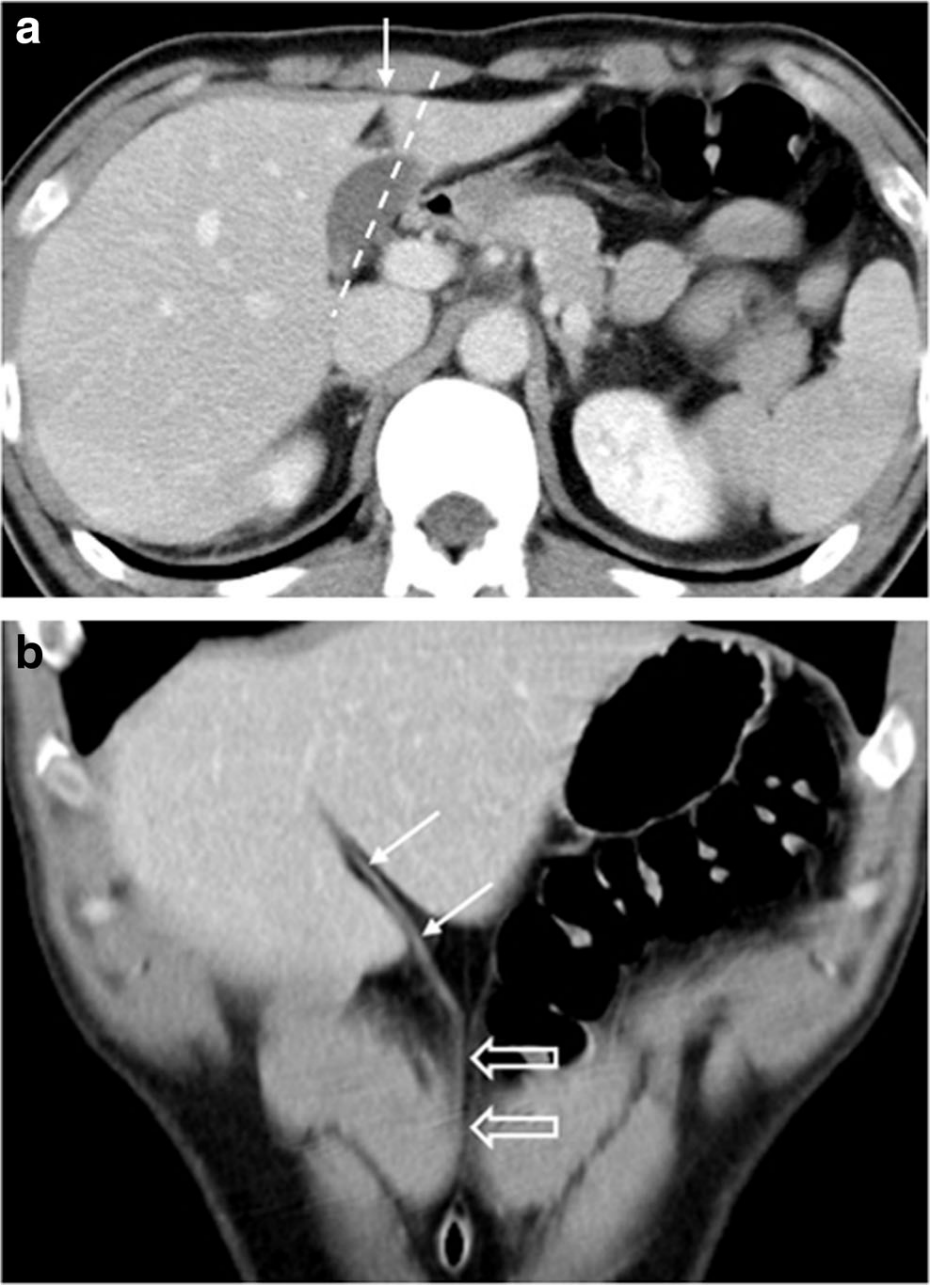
a Contrast-enhanced CT scan revealing the gallbladder’s cholecystic axis (*dotted line*) left of the umbilical fissure and RSLT (*arrow*). b The umbilical ligament (*thick arrows*) joined with the RSLT (*thin arrows*) is shown
